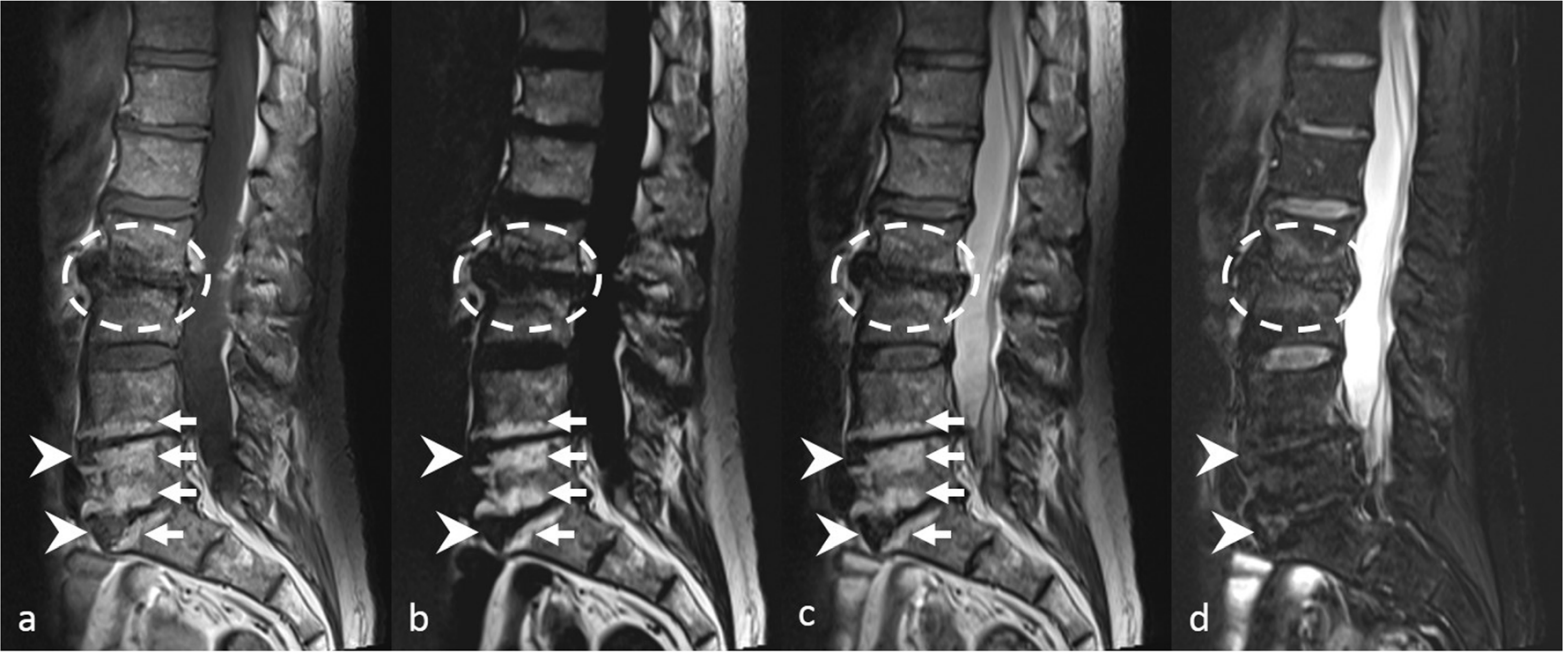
Lumbar spine MRI in a 63-year-old male including T1-weighted (a), fat-only image (b), in-phase image (c), and water-only image (d) from T2-weighted Dixon sequence. Fatty Modic type 2 changes are visible along the vertebral endplates adjacent to L4-L5 and L5-S1 discs on a–c (arrows). Degenerative changes at the anterior margins of vertebral bodies are also seen at the same levels (arrowheads). Mixed inflammatory and fatty Modic changes are seen along the vertebral endplates adjacent to L2-L3 disc (dashed circles). Note the high contrast between fatty changes and surrounding tissues on b
